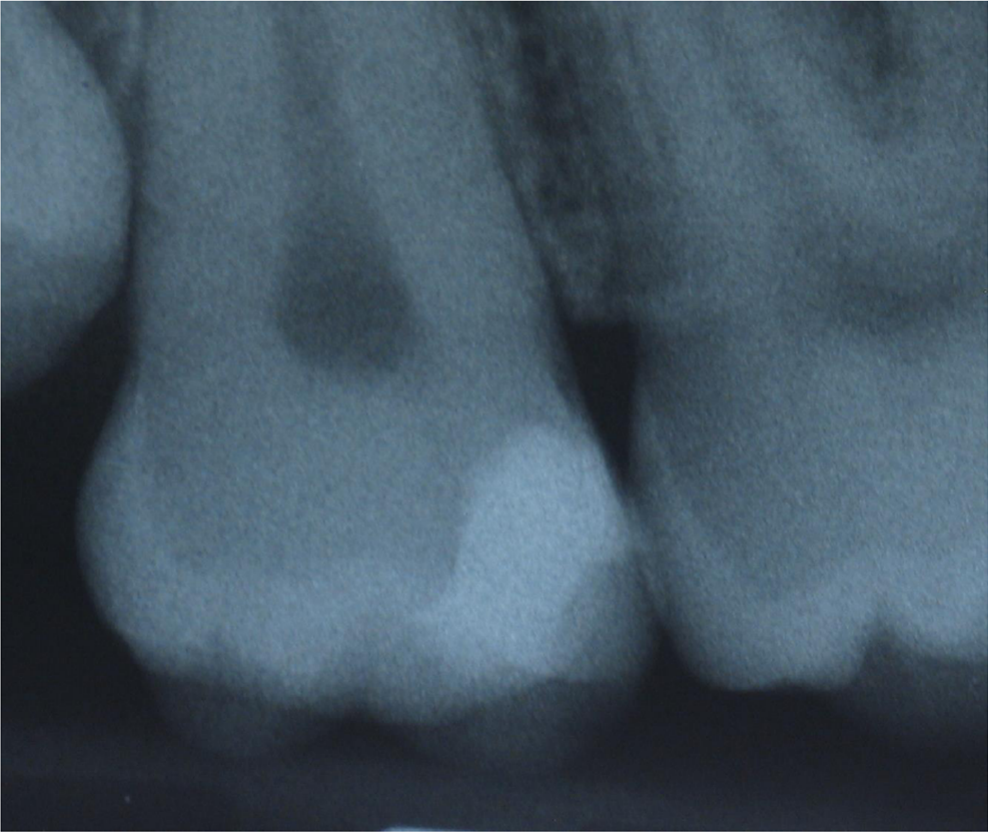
Corresponding radiograph of Figs. 8 and 9
